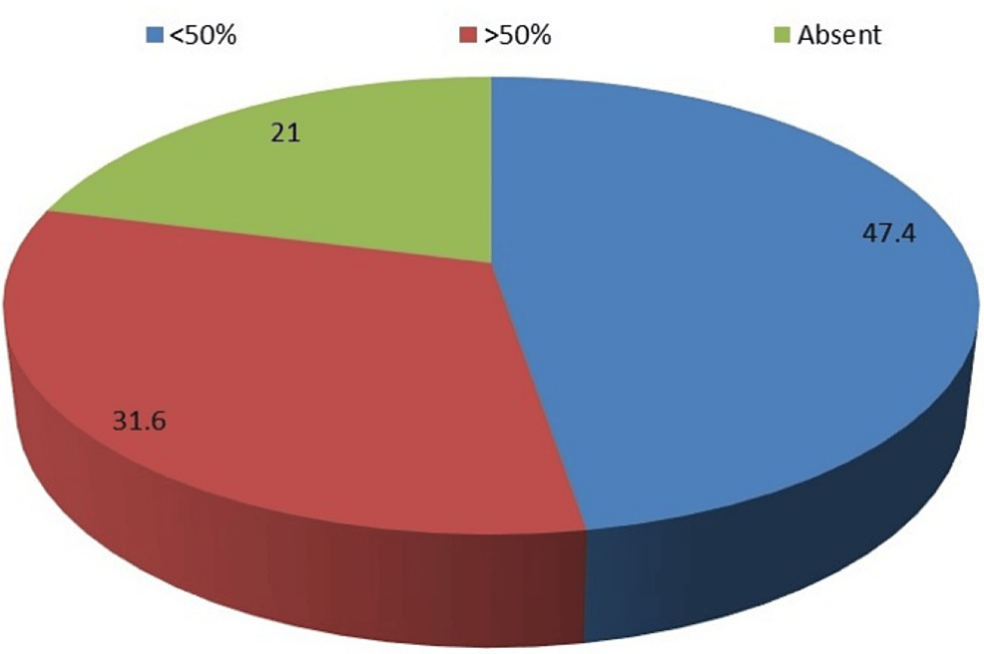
Distribution of myometrial infiltration on DCE-MRI DCE-MRI = dynamic contrast-enhanced MRI
